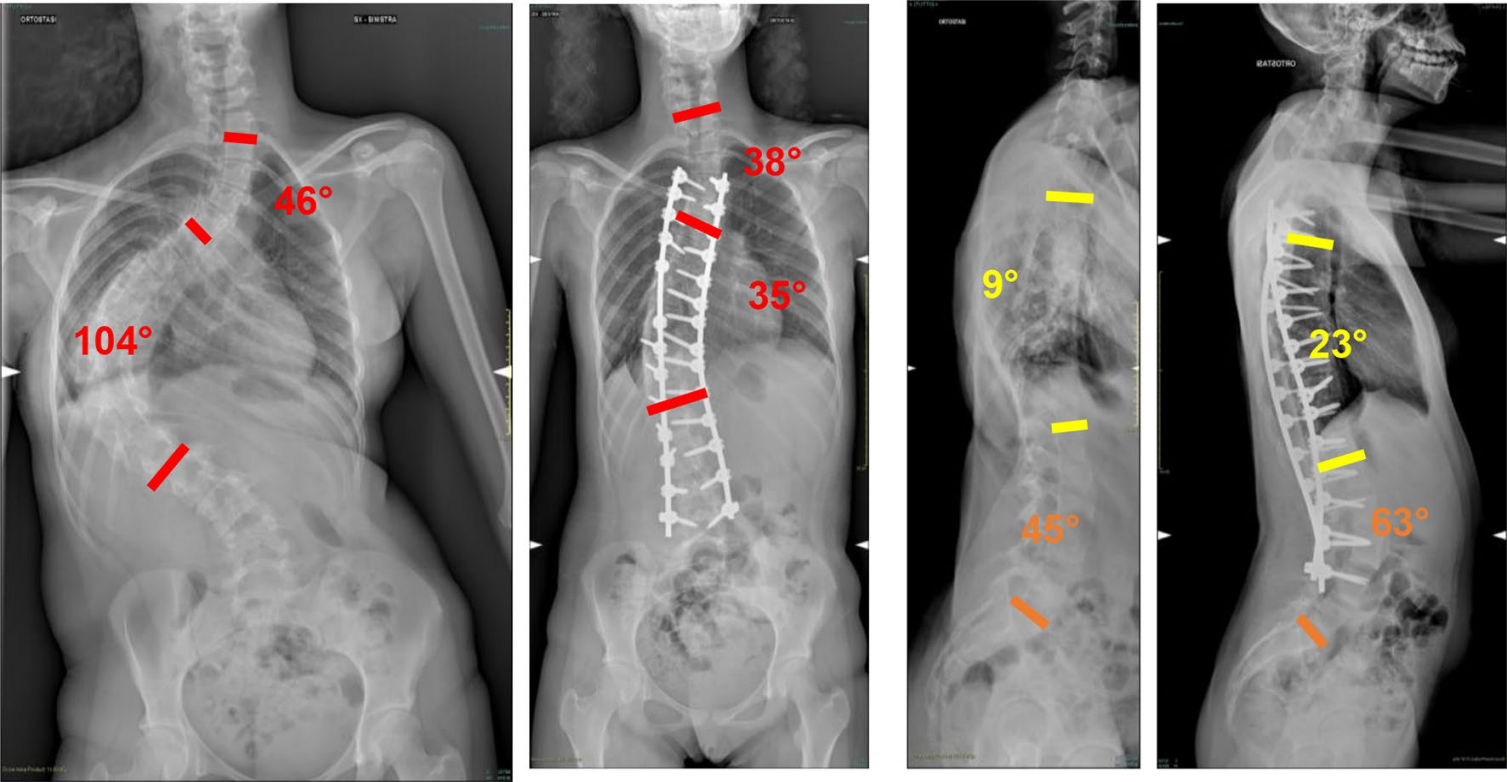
Case 1, a 17 years old female with a Lenke 2 curve, treated by T4–L4 fusion; pre and post-operative X-rays
